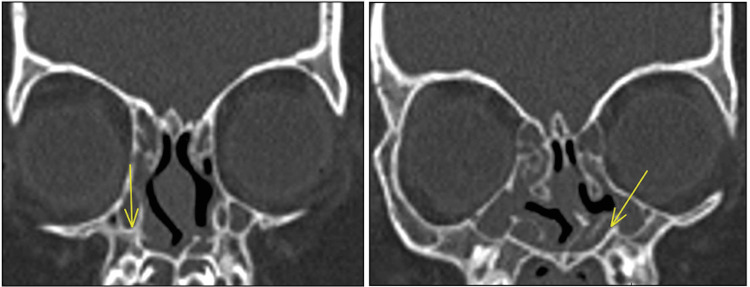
CT scan of the sinuses demonstrating complete bony stenosis at the distal ends of the bilateral nasolacrimal ducts in a patient with Saethre-Chotzen syndrome (arrows) CT: computed tomography
